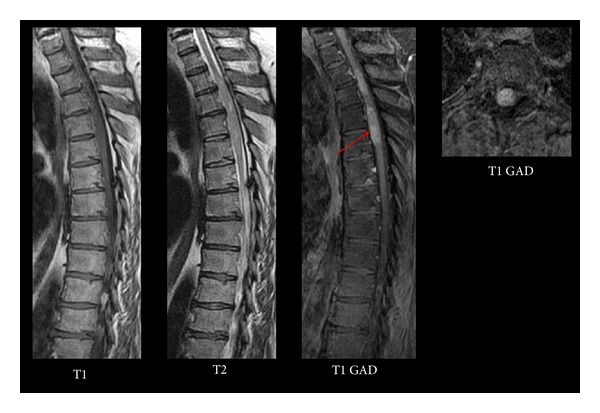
Preoperative thoracic MRI shows three sagittal sequences (T1, T2, and T1 with gadolinium) on the left and an axial (T1 with gadolinium) on the right demonstrating an intramedullary mass between T2 and T5 (arrow).
